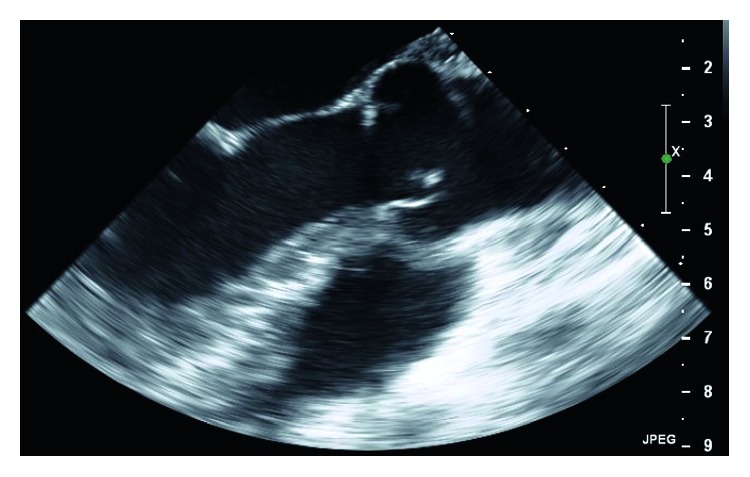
Follow-up transesophageal echocardiogram at 24 weeks showing a dramatic reduction in the size of the mass between the right and noncoronary cusps.
